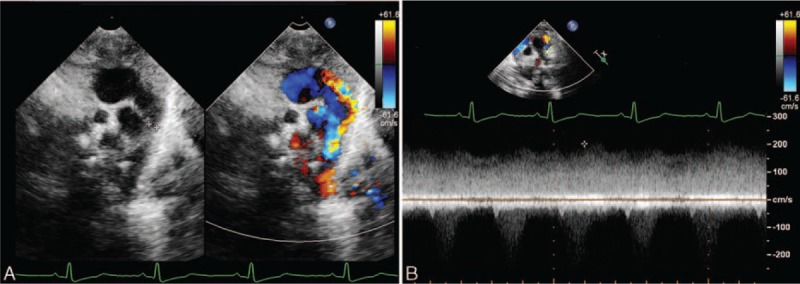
Color and pulse Doppler showing obstruction of the vertical vein in a 1-month-old girl with supracardiac total anomalous pulmonary venous connection. (A) Dual live image of 2-dimensional and flow Doppler showing turbulence flow of the obstruction. (B) Pulse Doppler shows the nonphasic flow of the stenosis.
